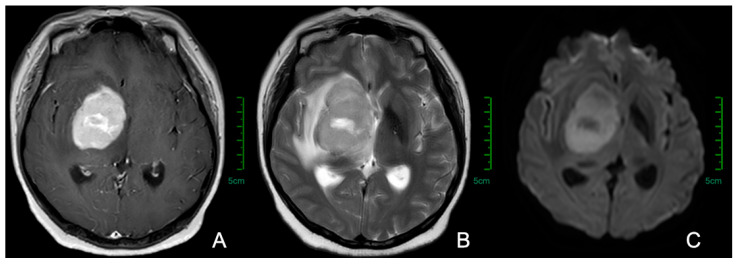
Characteristic imaging features of PCNSL on MRI. Magnetic resonance images from a patient with primary central nervous system lymphoma. (A) Contrast-enhanced T1-weighted sequence demonstrates homogeneous enhancement of the tumor in the right basal ganglia region. (B) T2-weighted sequence shows hyperintense signal surrounding the tumor, demonstrating vasogenic cerebral edema. (C) Diffusion-weighted imaging (DWI) demonstrates restricted diffusion within the lesions.
